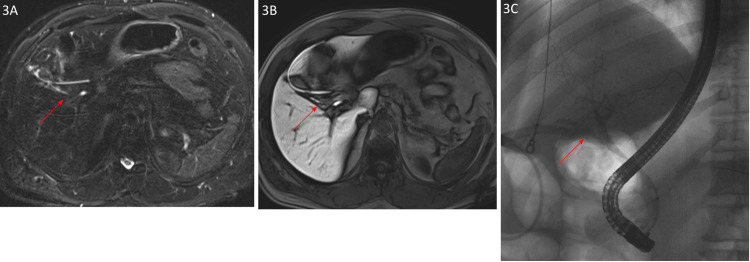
Imaging findings of a 57-year-old male: postoperative day 2 status post laparoscopic cholecystectomy (presenting with abdominal pain) MRCP demonstrates linear fluid collection in the gall bladder fossa extending from the cystic duct stump. Note the surgical drain in place (3A). One-hour delay axial vibe MRI image demonstrates linear enhancing fluid extending from the cystic duct stent, consistent with active biliary leak. (3B). ERCP image confirming active leak from the cystic duct stent (3C) MRCP: magnetic resonance cholangiopancreatography; MRI: magnetic resonance imaging; ERCP: endoscopic retrograde cholangiopancreatography
